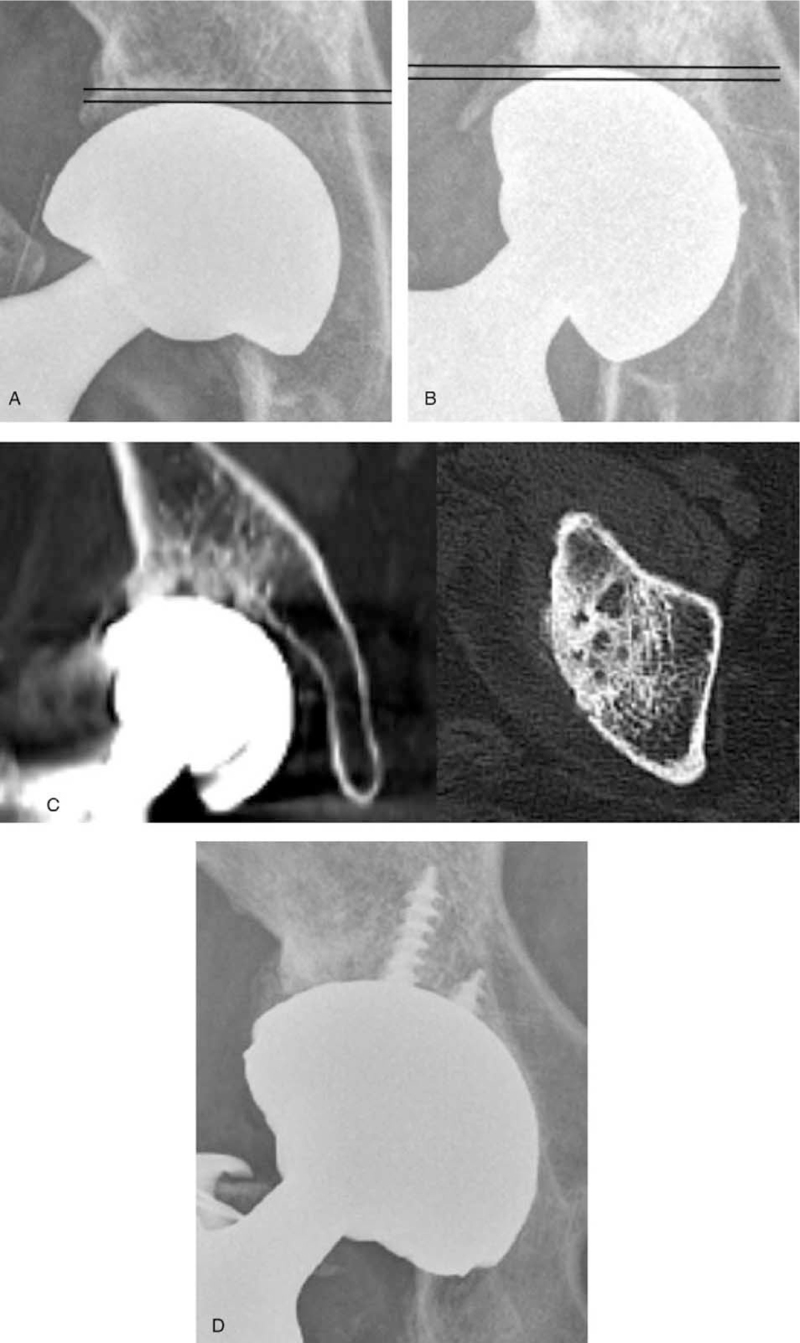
(A) A 80-year-old woman underwent bipolar hemiarthroplasty with femur neck fracture. (B) At 3 yr postoperatively, the bipolar cup was migrated superior up to the 3.8 mm. (C) Subchondral erosion with sclerotic change and cyst formation is seen on computed tomography. (D) She underwent conversion to total hip arthroplasty.
